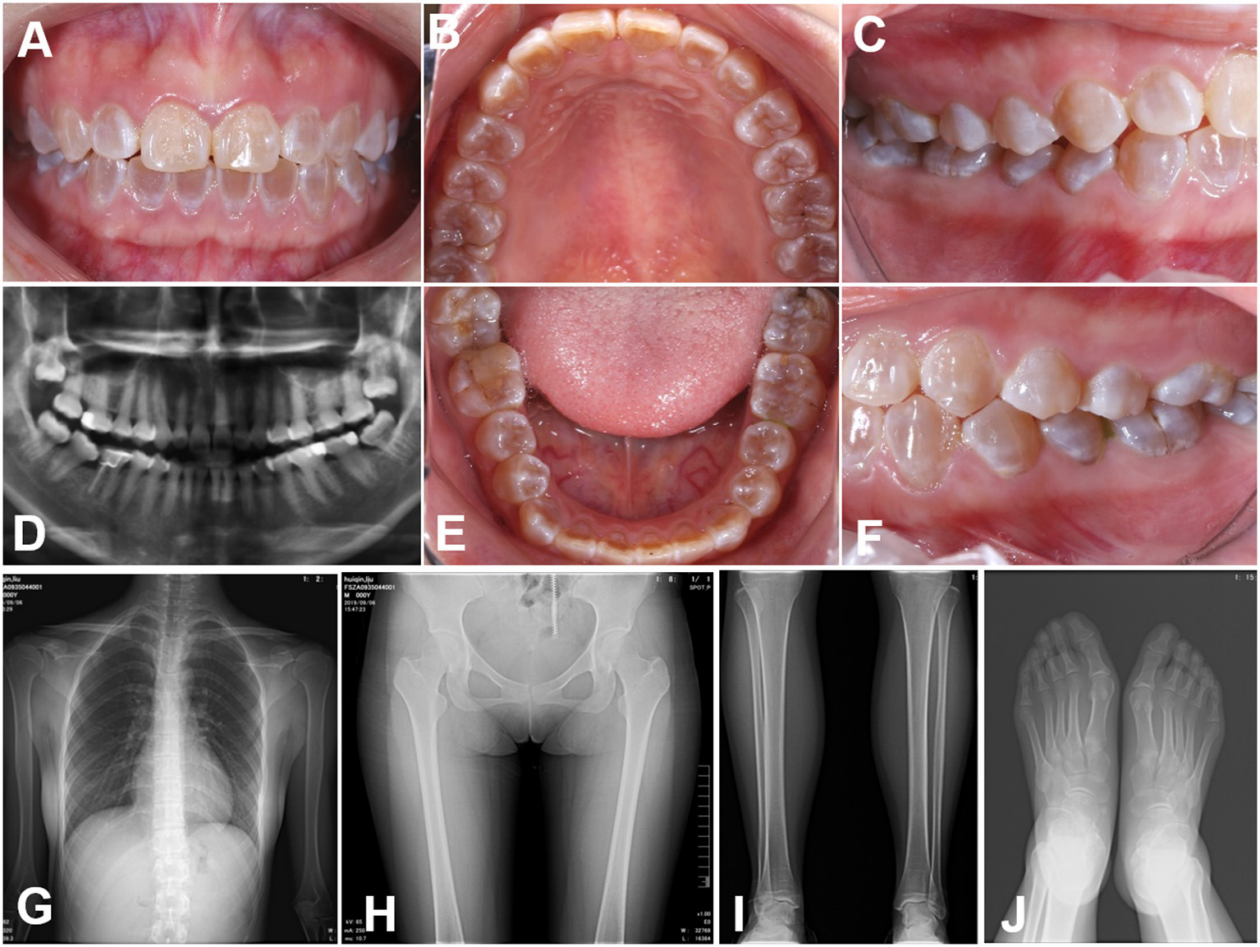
Clinical images (A–E) Intraoral views of the proband. The teeth of the proband were typically amber and translucent and showed significant attrition, especially the molar teeth. (F–J) Panoramic radiographs and radiovisiography images. The pulp chambers and root canals of the affected teeth were smaller than those in normal teeth or completely obliterated. Radiographs of the bones and knees revealed no significant osteopenia, bony destruction, periosteal reactions, or evidence of any acute fractures, dislocations or injuries.
